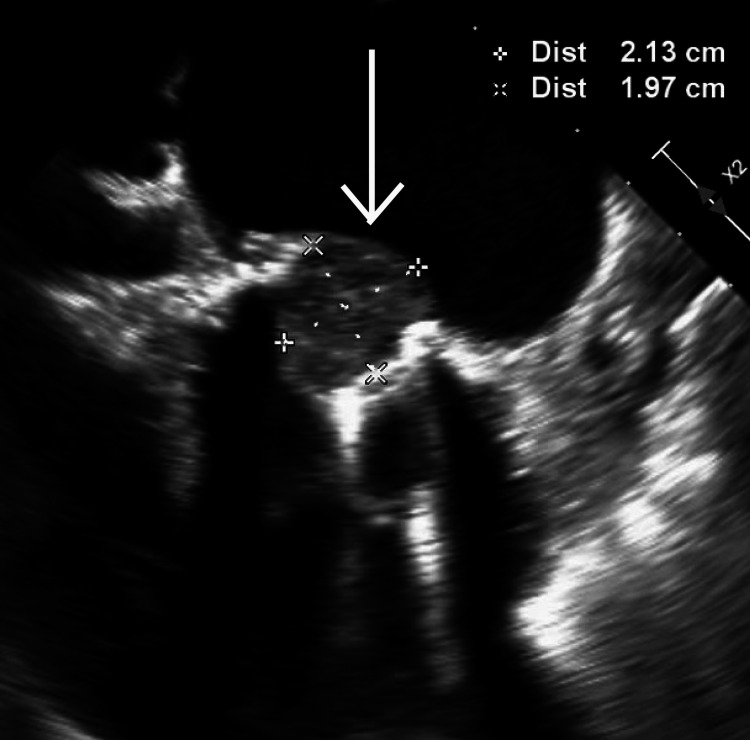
Transesophageal echocardiogram showing 2×2 cm thrombus on the left atrial side of the mechanical mitral valve
